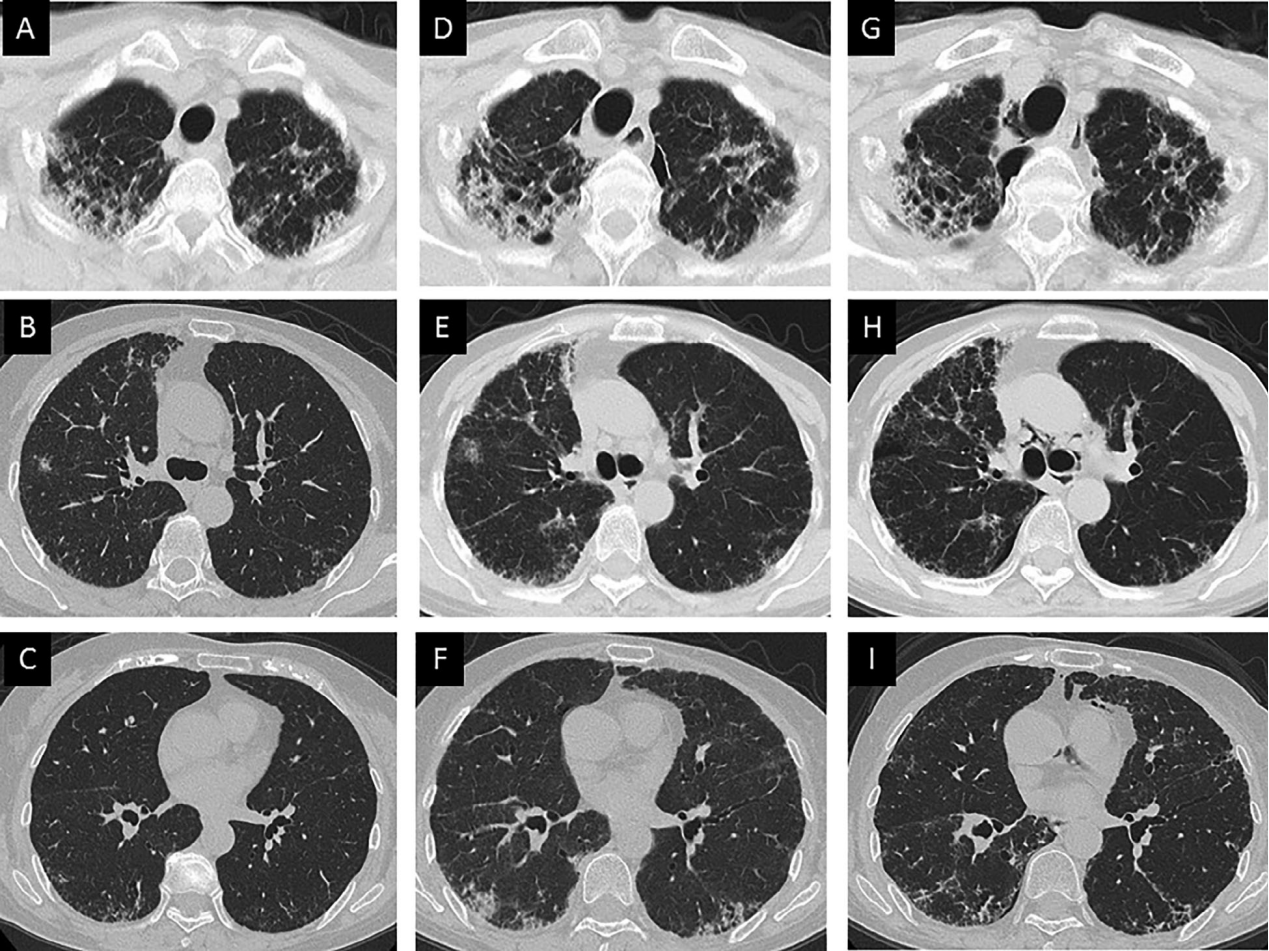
(A–C) Chest high‐resolution computed tomography (HRCT) images revealed patchy bilateral ground‐glass opacities (GGO), nodular areas of consolidation, and multiple small nodules in the bilateral upper lobes predominance, in addition to bilateral dense subpleural consolidation and irregular septal thickening at the level of the lung apices. (D–F) Twelve months after the initial visit, reticulonodular lesions in both lung and coarse fibrosis predominating in the peripheral upper lobes worsened. (G–I) Three months after treatment with corticosteroid and cyclosporine, reticulonodular lesions in both lung and bilateral subpleural reticulation at the level of the lung apices were partially improved. Pneumomediastinum and minor pneumothorax were seen.
